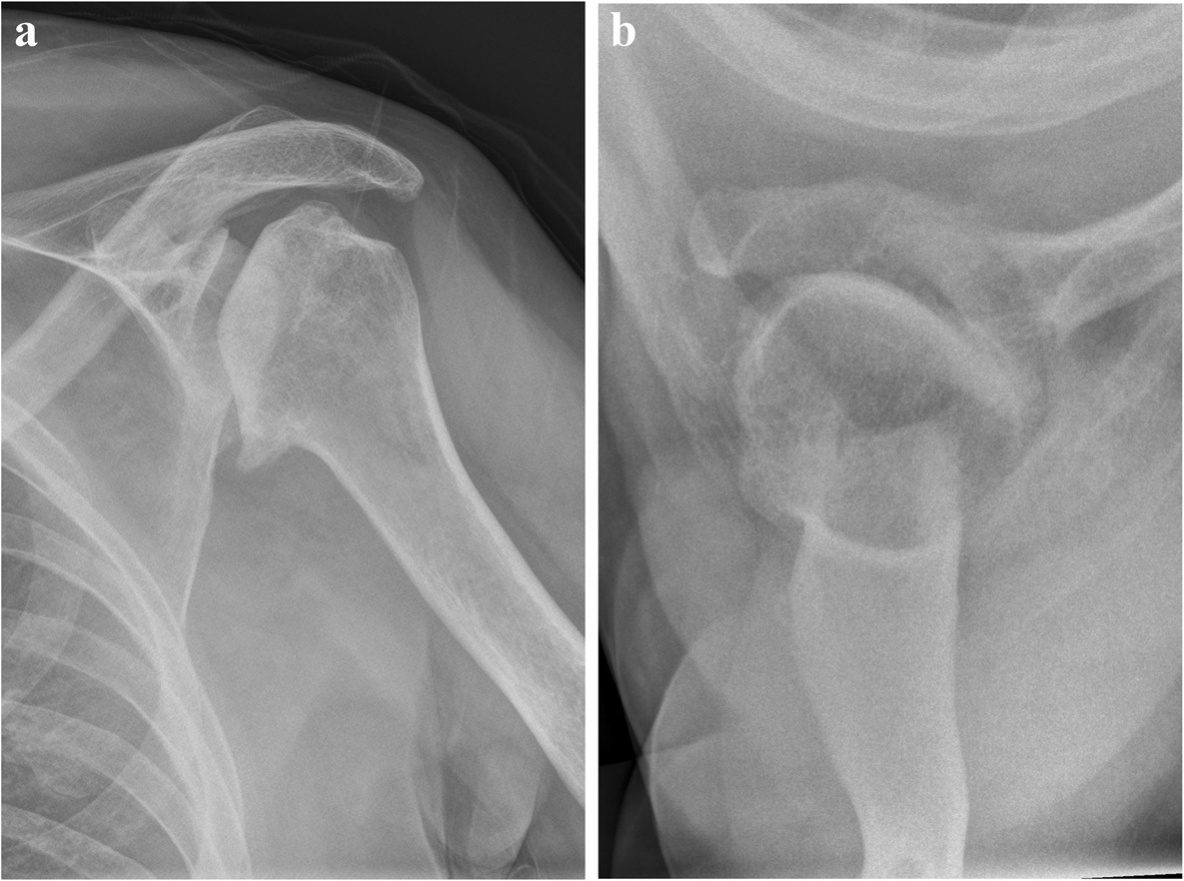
a and b: Preoperative true AP and axial view of the left shoulder show the displacement of the joint and hypoplasia of the glenoid. X-rays reveal a small, irregularly shaped bone (Case 3). Poor ROM prevents optimal visualization of the bone stock and the glenoid surface
